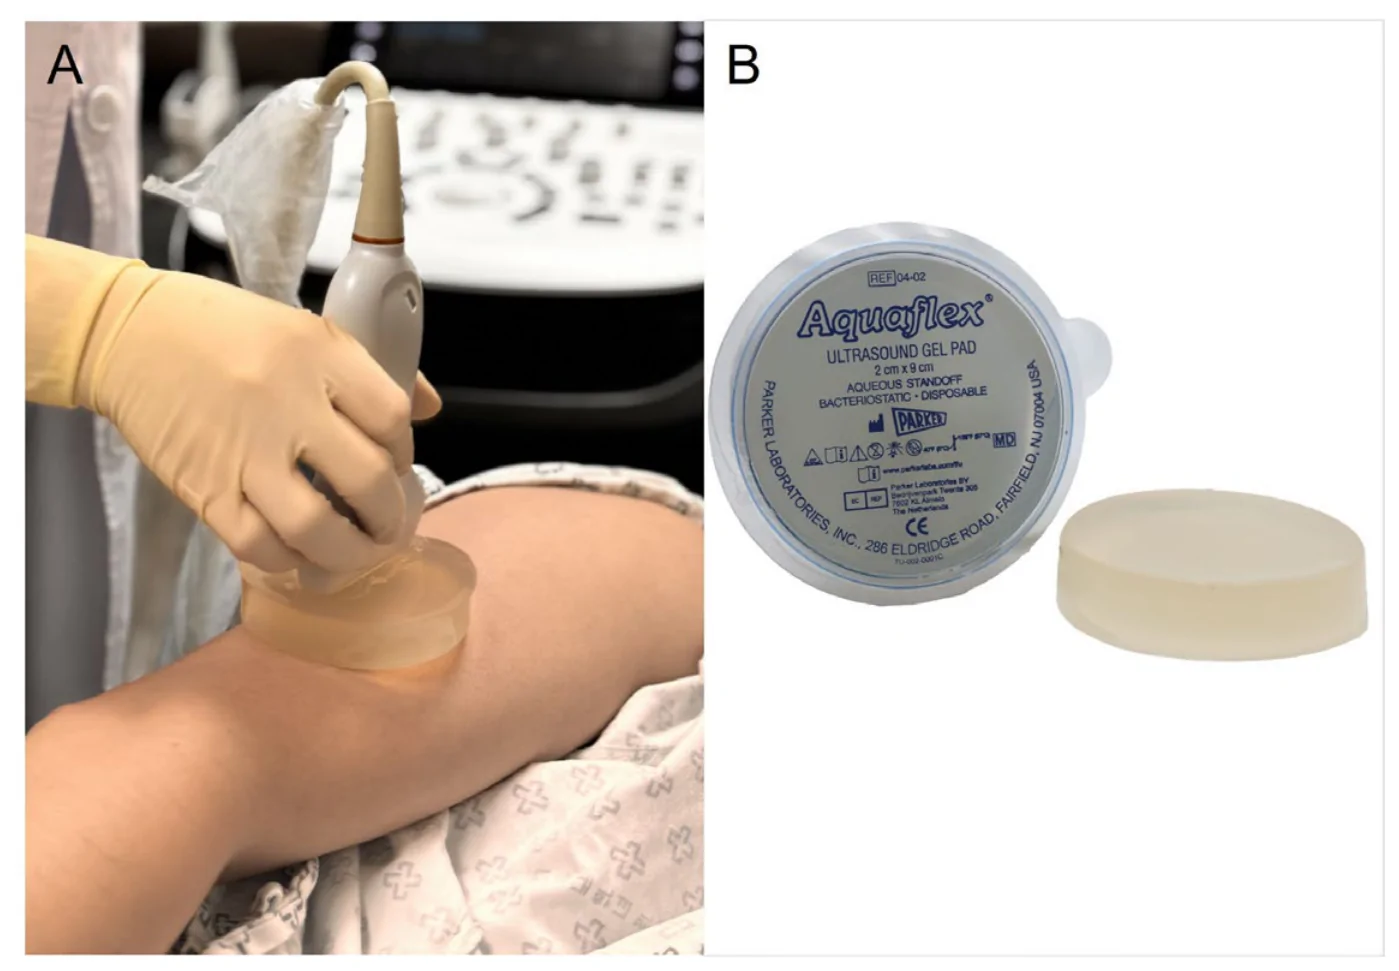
Ultrasound and shear wave elastography examination protocol. (A) Measurements were performed with the participant in the lateral decubitus position. The upper limb was assessed at sites located 10 cm proximal and 10 cm distal to the elbow crease. An Aquaflex ultrasound gel pad (Parker Laboratories) was used to minimize tissue compression caused by transducer pressure. (B) The Aquaflex ultrasound gel pad (Parker Laboratories) used during all ultrasound and shear wave elastography examinations.
